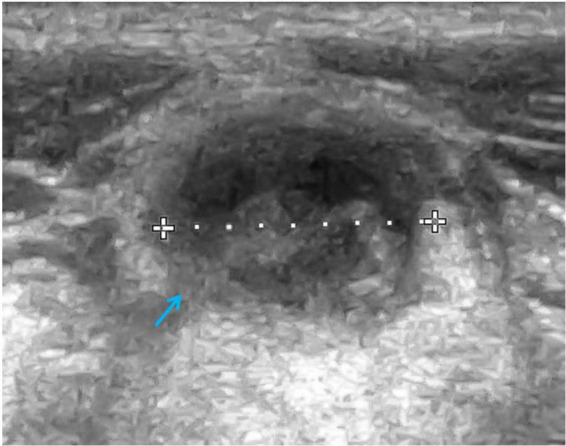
Ultrasound (US) examination of the lymph node, linear probe. A cervical lymph node with an abnormal echostructure, heterogeneous hypoechogenicity, and change of a shape from oval to round (marked with an arrow).
